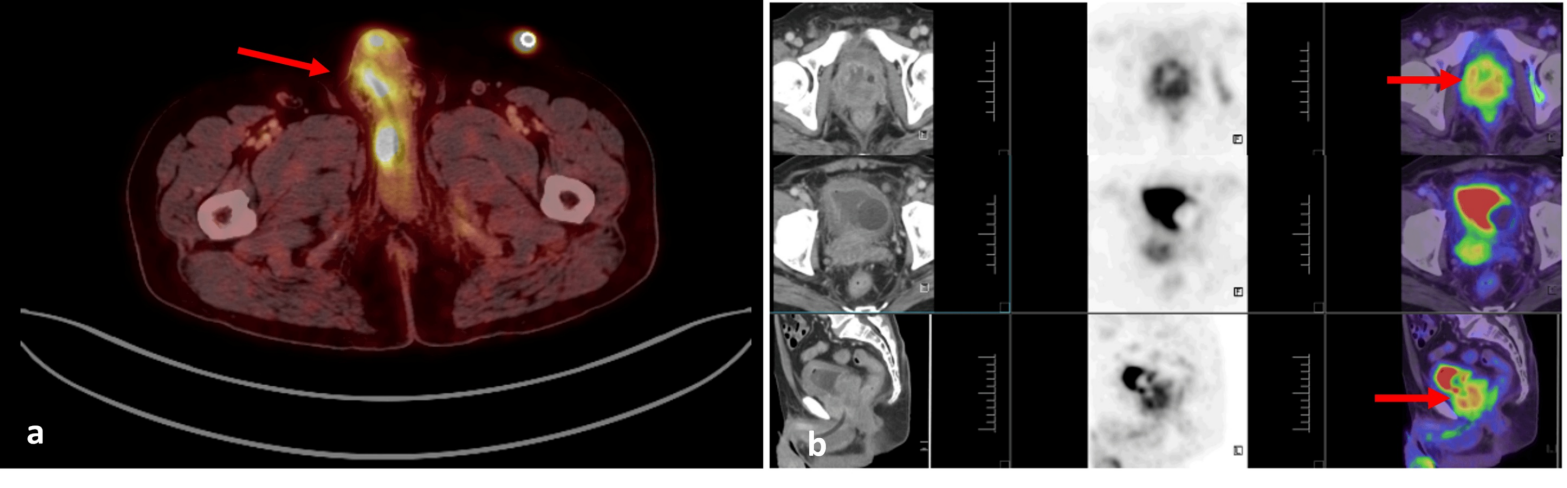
Positron emission tomography showing FDG avid penile lesions Multiple metabolically active FDG avid lesions in the penis (suggestive of metastasis) (a), malignant-looking mass lesion seen replacing the prostate and involving bilateral seminal vesicles and trigone of the urinary bladder (b).
